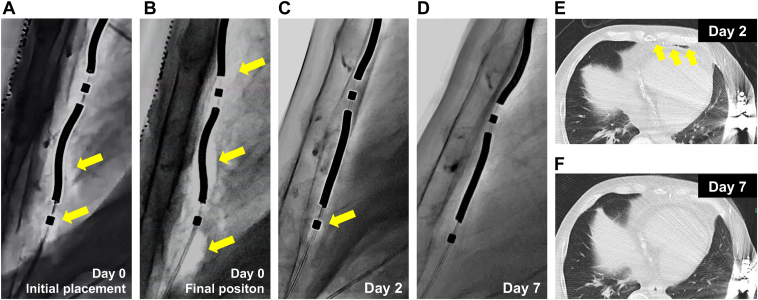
Resolution of air entrapment around the substernal lead. Serial lateral fluoroscopic views show (A) at the initial position, (B) at the end of the index operation, (C) on postoperative day 2, and (D) 1 week after implantation. Entrapped air surrounding the ring 1 and ring 2 electrodes progressively decreased over time and resolved completely by 1 week. Radiolucent areas indicating entrapped air are highlighted with *yellow arrows*. Computed tomography (E) on postoperative day 2 demonstrated residual entrapped air surrounding the substernal lead, (F) whereas repeat imaging on postoperative day 7 confirmed complete resolution of the air entrapment.
